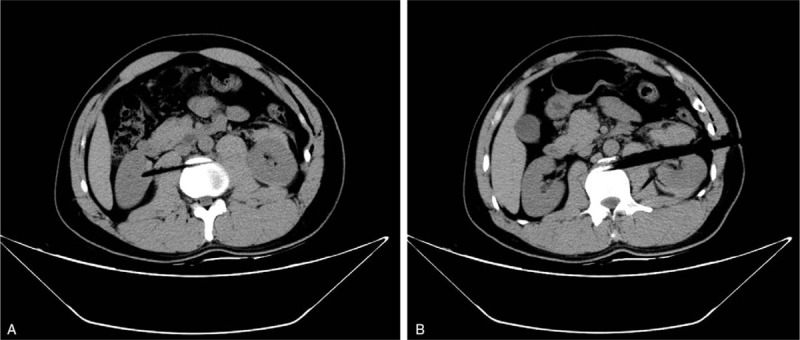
Preoperative CT scan (A: The stick went through the right psoas muscle and terminated in the right renal parenchyma. B: The stick went through the left kidney, the left psoas muscle and the anterior body of the second lumbar vertebra.)
